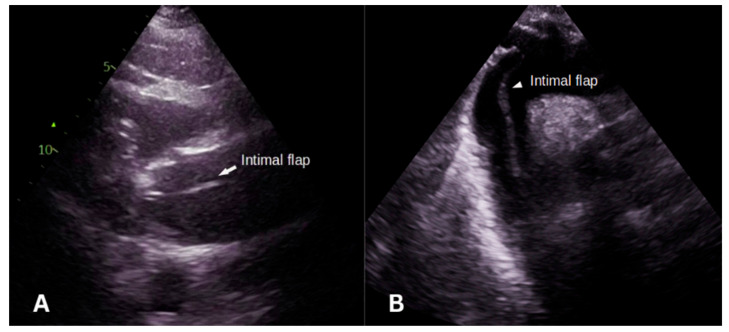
Acute aortic dissection with intimal flap (white arrow). (A) Parasternal long axis view; (B) suprasternal view of aortic arch (image courtesy of E. Kiouri and E. Polyzogopoulou).
